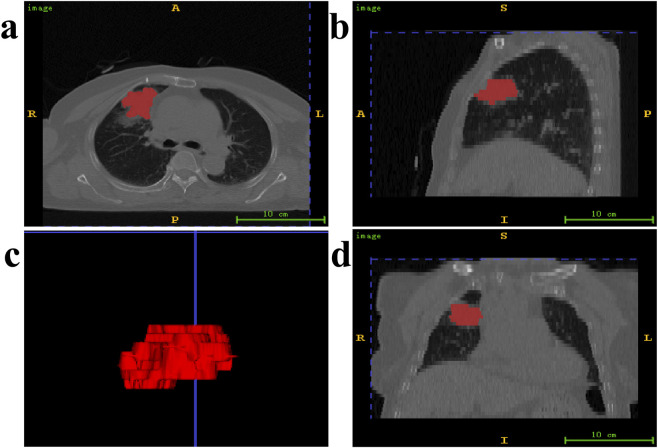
A CT image with the mask overlay for tumor segmentation: (a) axial view, (b) sagittal view, (c) 3D view, (d) coronal view.
